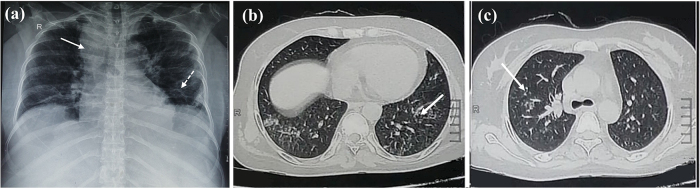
(a) Chest x-ray shows the right upper lobe nodules (solid white arrow) and reticulonodular shadowing mainly in the left lower lobe (white dotted arrow). (b) CT chest showing bilateral reticulonodular shadowing mainly in the left lower lobe, represented with white arrows. (c) CT chest demonstrates ground glass opacities, mainly in the right upper lobe, represented with a white arrow.
